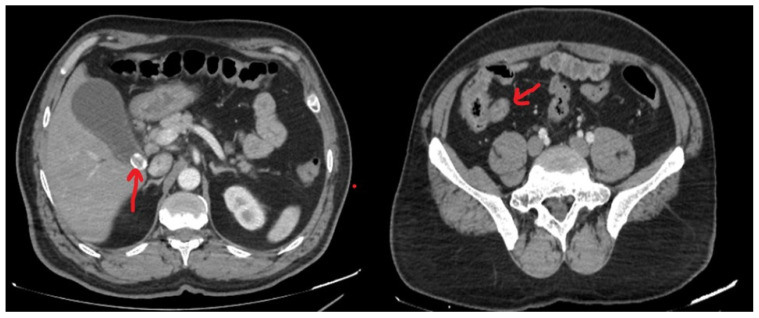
Axial computed tomography (CT) images of the same patient. The left image demonstrates a gallstone obstructing the cystic duct, gallbladder wall thickening, and a hydropic gallbladder. The right image reveals radiologic features consistent with AAP (The red arrow in the left image points to the gallstone located within the hydropic gallbladder, while the red arrow in the right image indicates the vermiform appendix).
